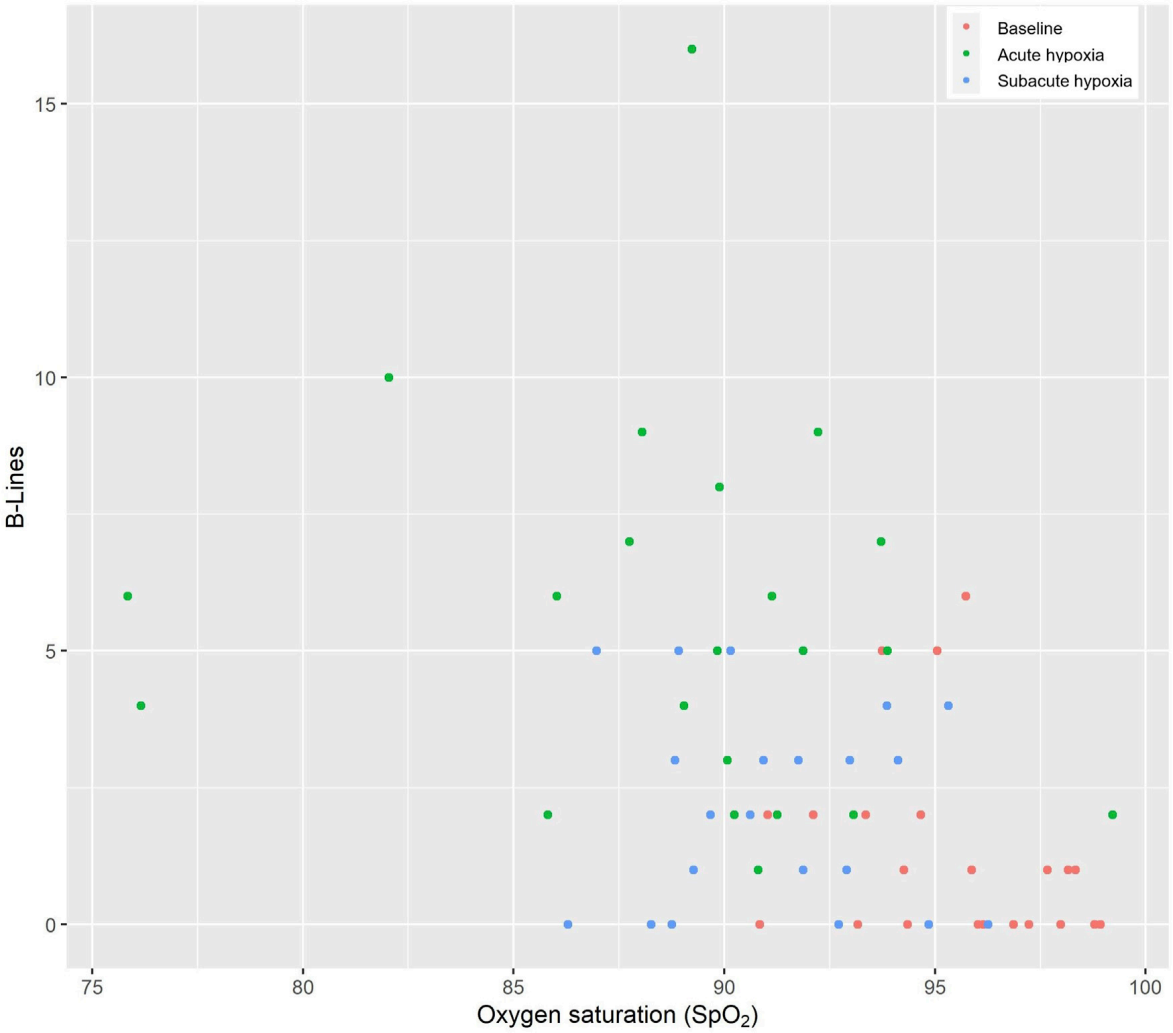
Thoraxsonography of the extravascular lung water (B-lines) and the oxygen saturation. The red circles indicate the baseline values at low altitude, the green circles indicate acute hypoxia, and the blue circles indicate subacute hypoxia.
